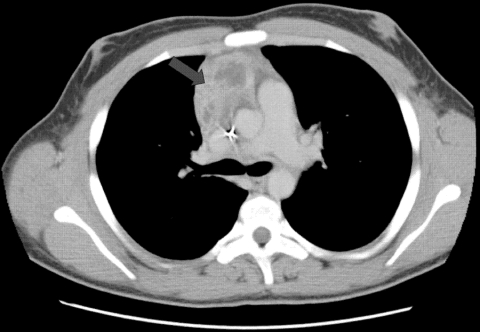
Computed tomographic scan of thorax showing extension of infection with *Aspergillus*
